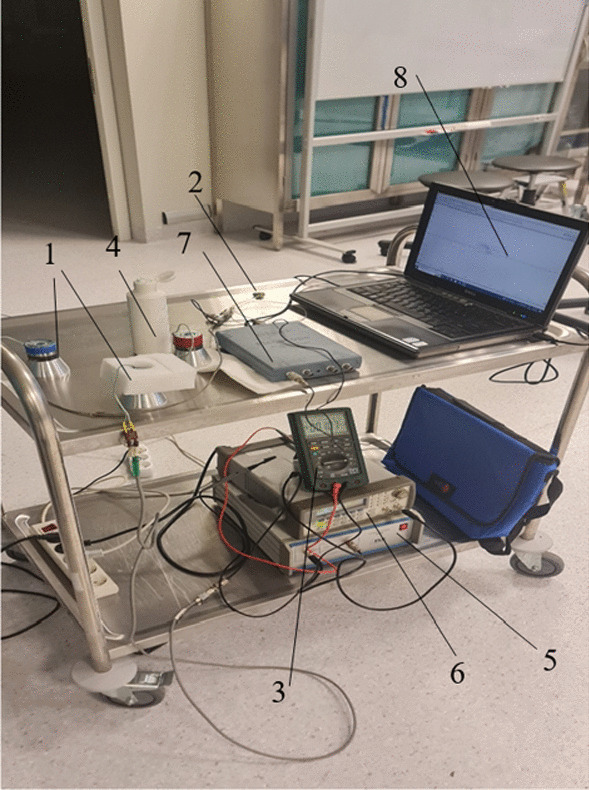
Experimental setup for measurement of the sonication intensity transmitted through tissue by an ultrasonic transducer: 1—ultrasonic transducer; 2—hydrophone; 3—RMS multimeter MS8218 (Mastech Group Ltd.); 4—ultrasonic gel Transonic G-15 (TELIC, S.A.U., Spain); 5—power amplifier P200 (FLC Electronics AB, Sweden); 6—signal generator Agilent 33220A; 7—oscilloscope PicoScope 3424 (PicoTechnology Ltd., UK); 8—PC
